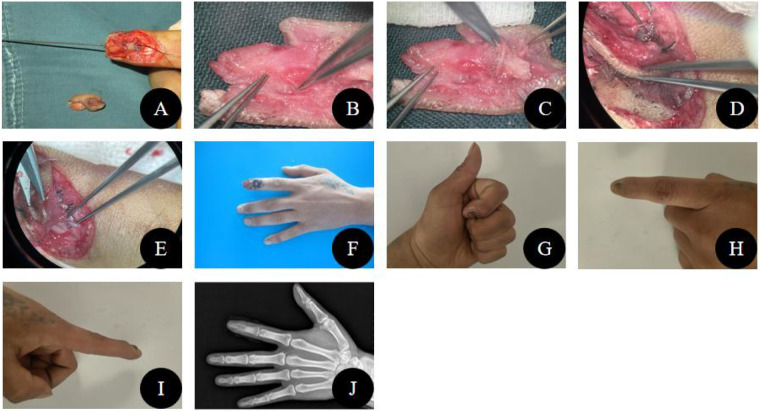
Case of arterial reconstruction using the proximal subungual arch artery as an alternative to the palmar digital artery. (A) Amputation of the tissue flap on the dorsal side of the left index finger's distal phalanx. (B) The proximal subungual artery on the radial side was identified during surgery. (C) The dorsal subcutaneous vein was identified during surgery. (D) Intraoperative view showing anastomosis of the proximal subungual arch artery and the nail bed nerve on the radial side to the palmar digital artery and nerve, respectively. (E) One dorsal subcutaneous vein was anastomosed during surgery. (F) Survival of the replanted tissue. (G–I) Nail growth was observed during the 16-month postoperative follow-up. (J) An x-ray taken two months after surgery showed that the fracture of the left index finger had healed well, and the internal fixation was removed.
